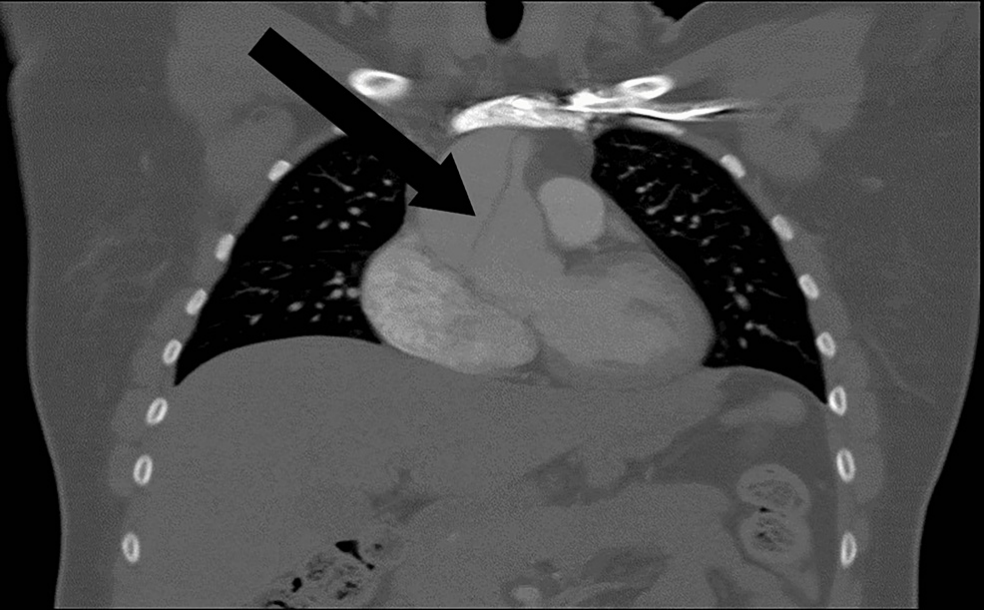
CT coronal image of the ascending aortic aneurysm dissection (black arrow) CT: computed tomography
